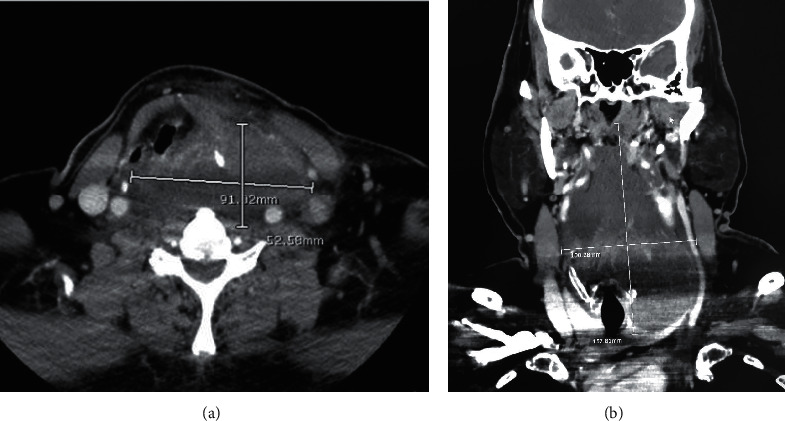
Computed tomography scan of the neck. (a) Axial and (b) coronal views showing a large lobulated partial rim-enhancing hypodense lesion with internal septation in the deep space of the left neck.
